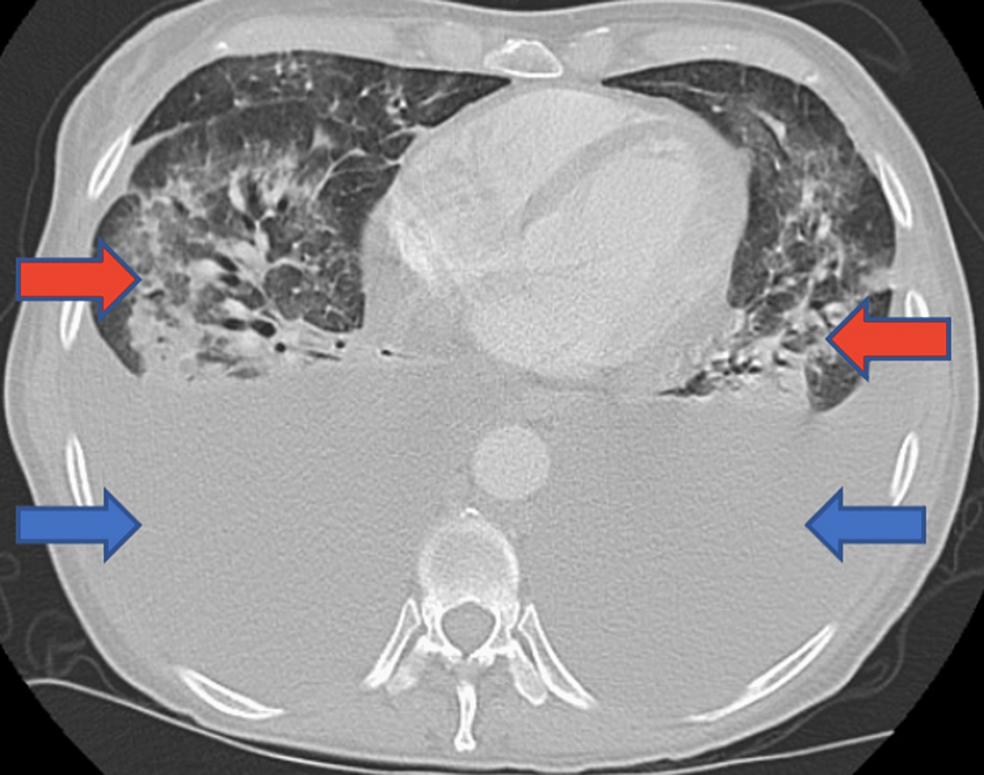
CT of the chest on the day of admission Blue arrows indicate large bilateral pleural effusions; Red arrows indicate severe bilateral pneumonia
